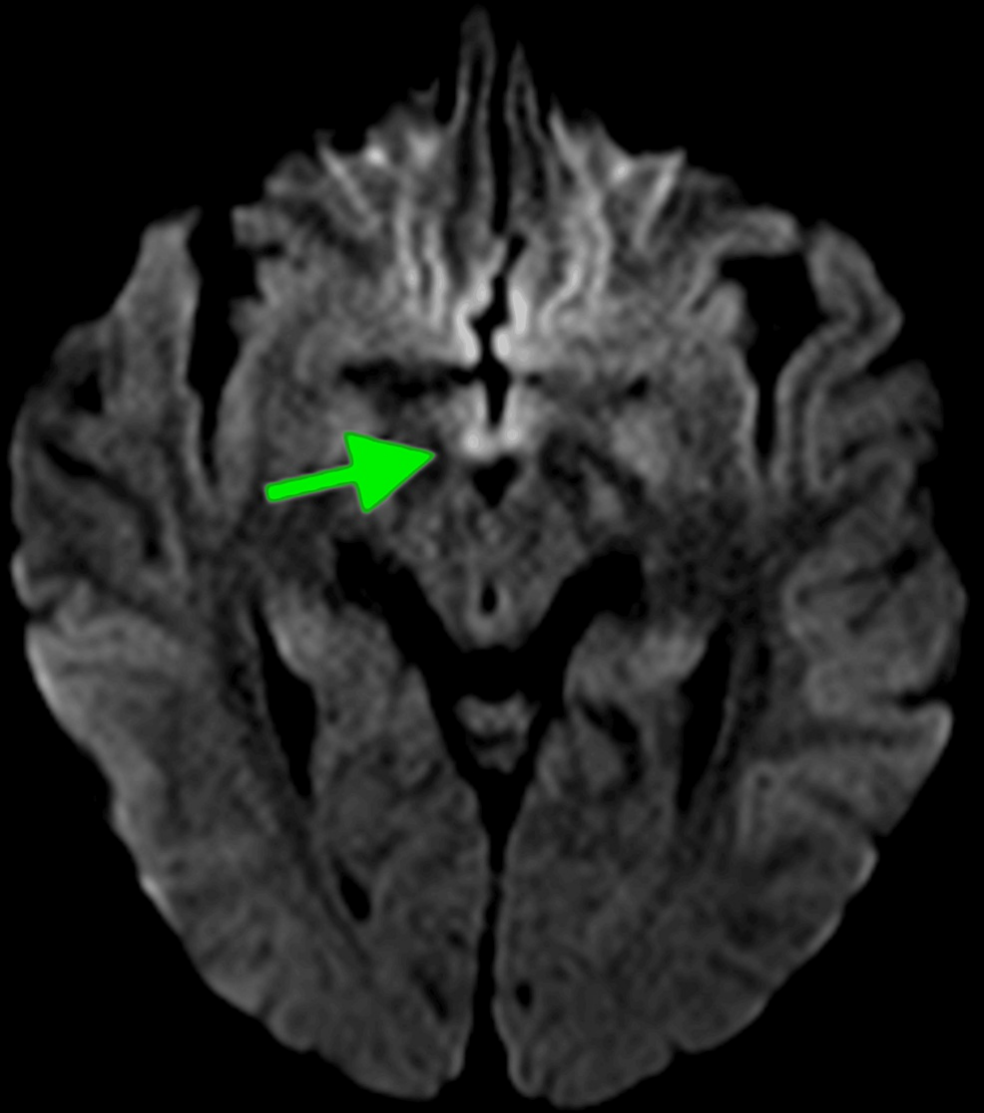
MRI brain with T2 imaging revealing edematous mammillary bodies (green arrow)
